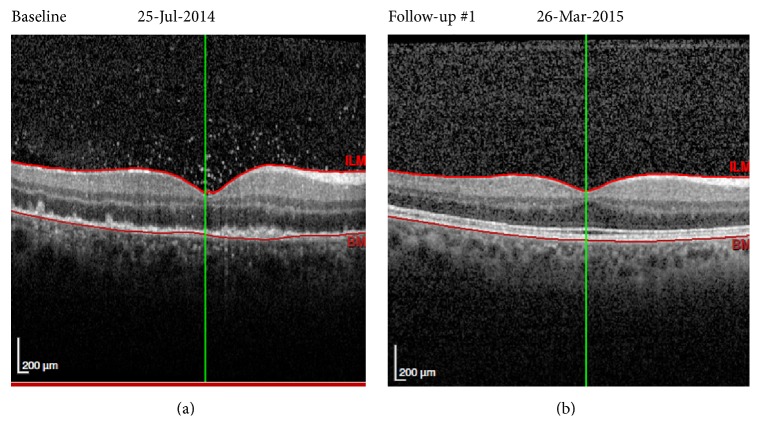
Macular optic computed tomography of right eye, during active disease (a). Small vitreous inflammatory agglomerates and inflammatory deposits in neurossensorial retina; diffuse macular thickening. After resolution (b).
